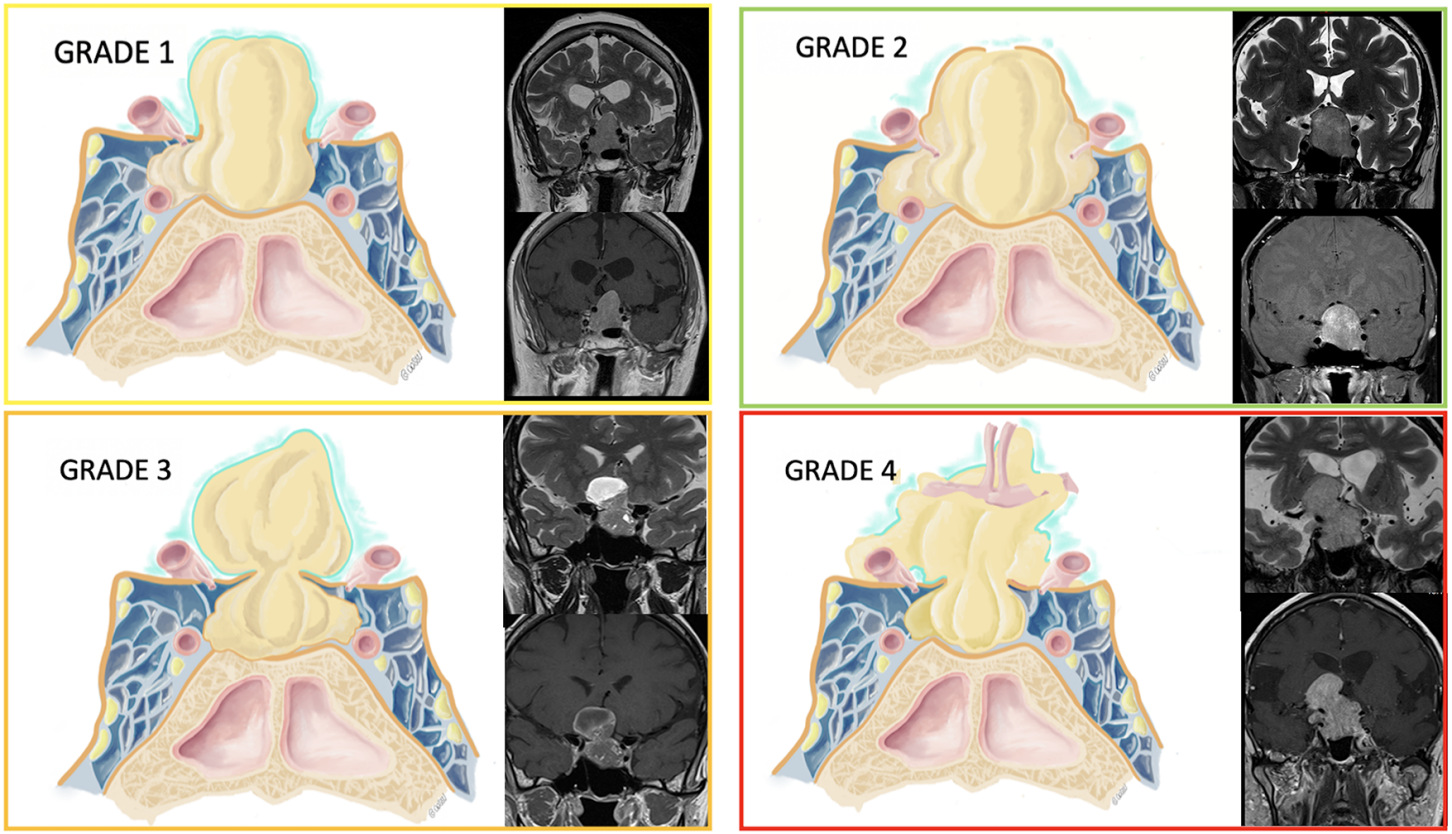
Tumors were classified according to their relationship with the diaphragm. A graphical representation is provided along with some examples of patients' MRI on the coronal plane (T2- and T1-weighted sequences after gadolinium injection).
